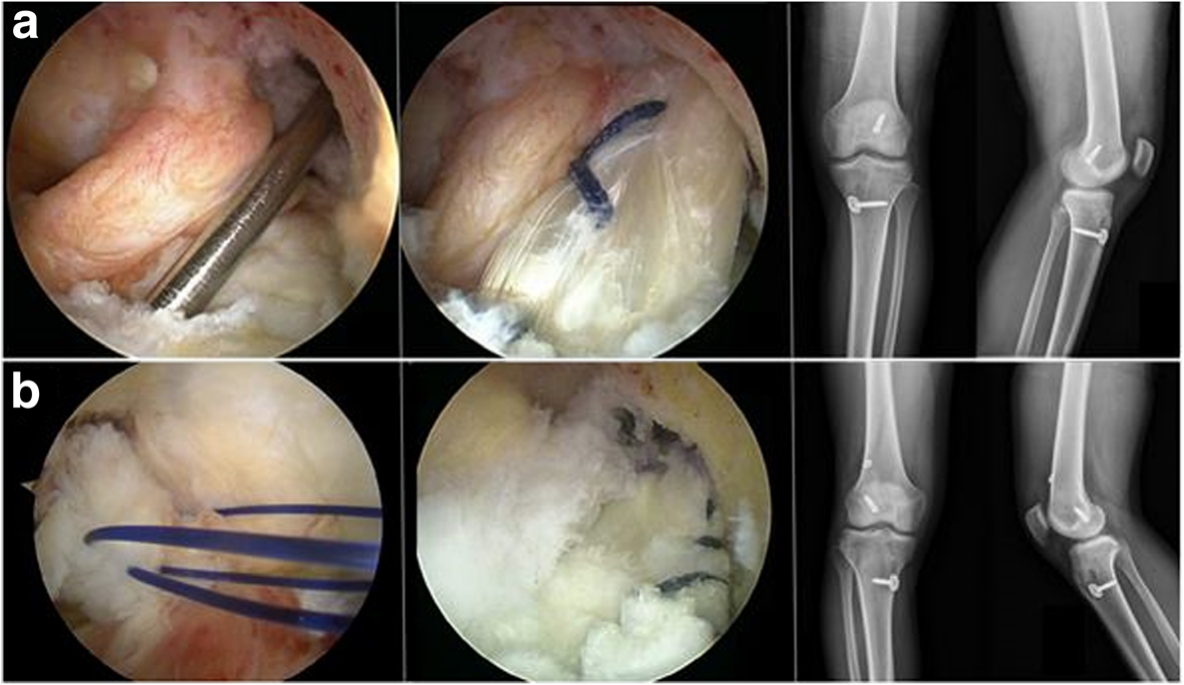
Arthroscopic findings for both types of preservation technique with postoperative radiography. a The remnant preservation technique with Achilles allograft reconstruction. b The remnant suture and tensioning technique with Achilles allograft reconstruction
